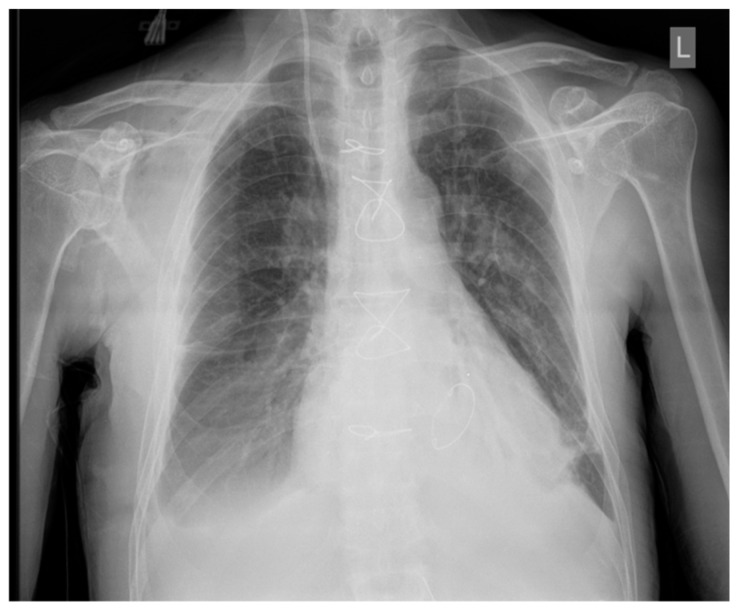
Chest X-ray at 3 days of VV-ECMO, suggestive of a favorable outcome of the alveolar infiltrates.
